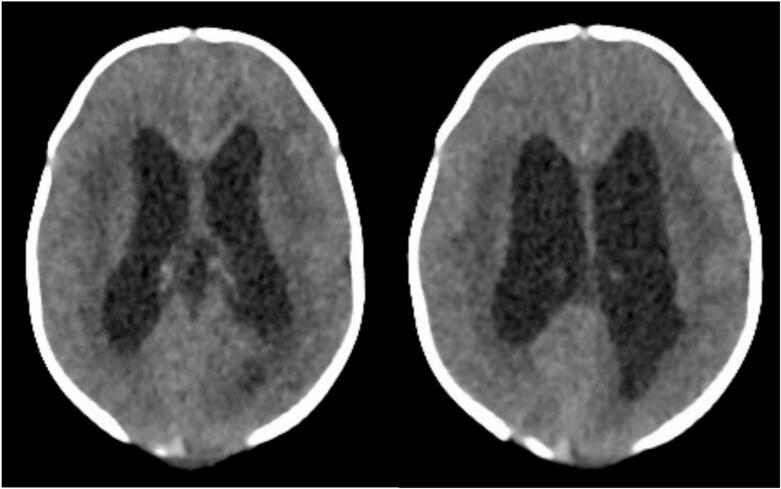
Non-contrasted volumetric CT imaging of the head from the base of skull to the vertex viewed in appropriate reformats and window settings: Moderately dilated lateral and third ventricle (severity has increased in comparison to previous MRI of brain). The fourth ventricle is not dilated. The occipital bone defect is noted with only fat contents within the herniated sac, no brain tissue seen. Persistent diffuse loss of sulcations noted. Corpus callosum not clearly defined.
